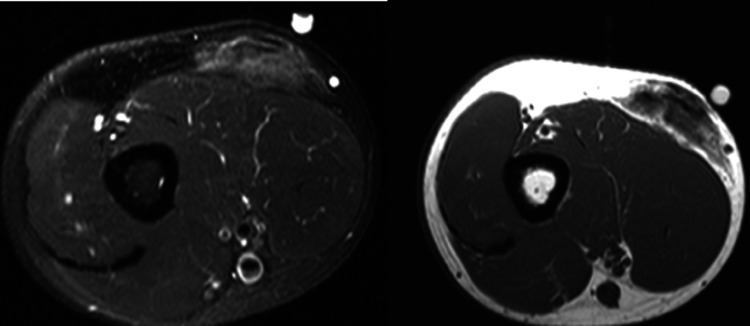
MRI of the upper extremity with and without contrast This image demonstrates a deep subcutaneous plane soft tissue mass located on the fascia lateral biceps mid-forearm. It shows faint T2 heterogeneous hyperintensity, with small foci of decreased signal possibly fibrosis, and less likely calcification. There is no invasion of deep fascia or muscle. Faint postcontrast enhancement was observed. Dimensions are approximately 1.6 x 3.8 x 4.6 cm (anteroposterior (AP) x transverse (TR) x craniocaudal (CC).
